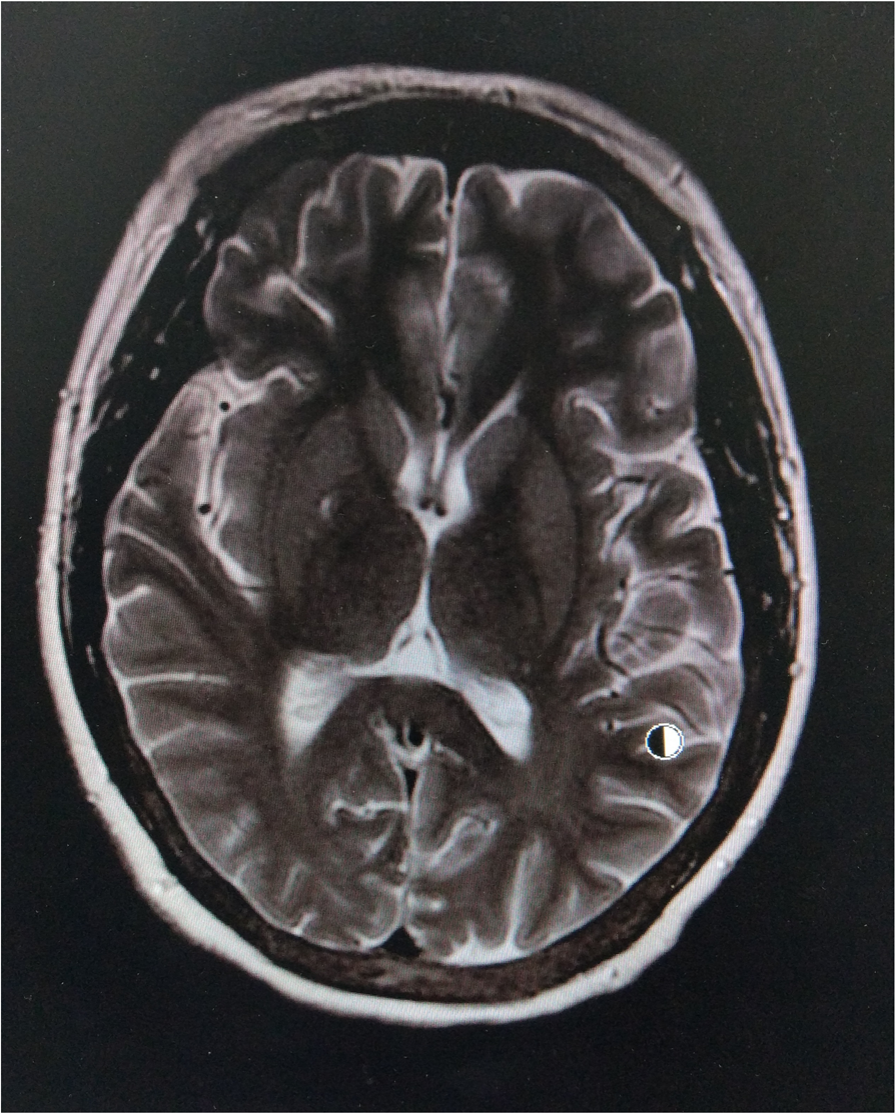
Follow-up MRI scan obtained after 1 month. T2WI showed almost complete resolution of the lesions with a slightly hyperintense signal
